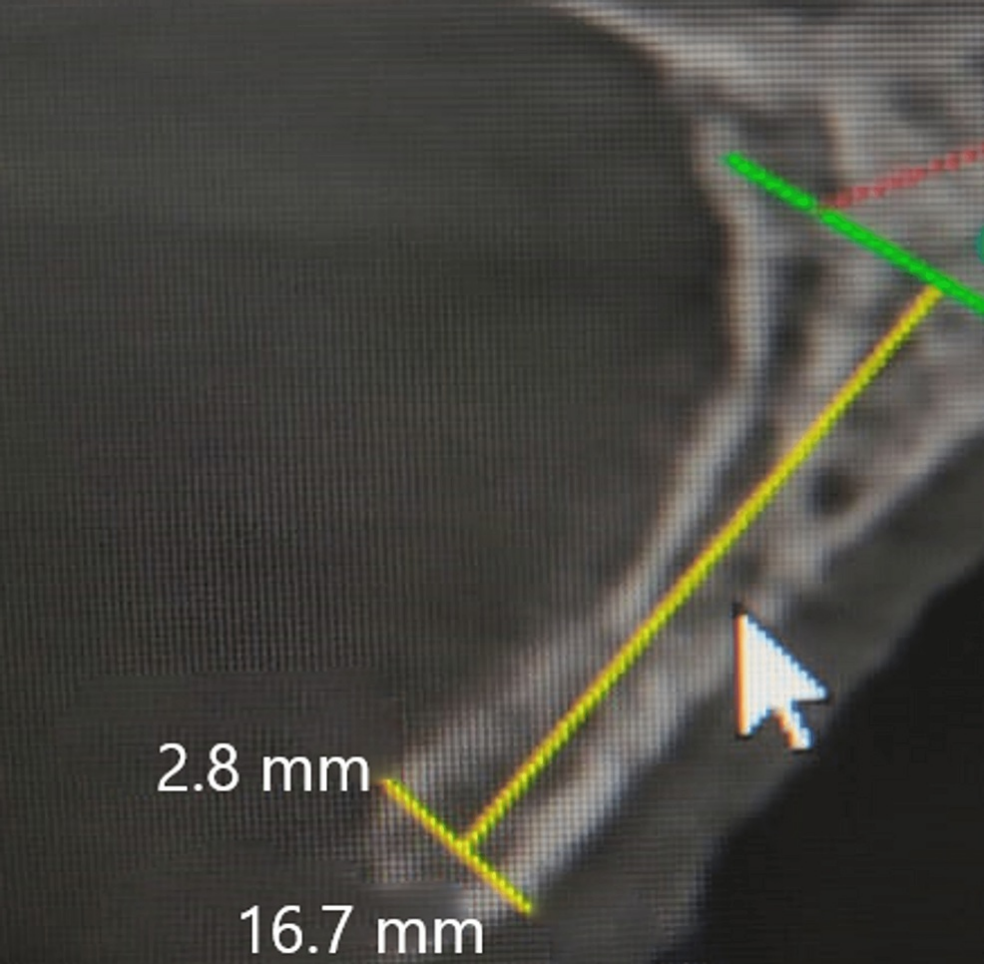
Preoperative CBCT of the patient CBCT: Cone-beam computed tomography
